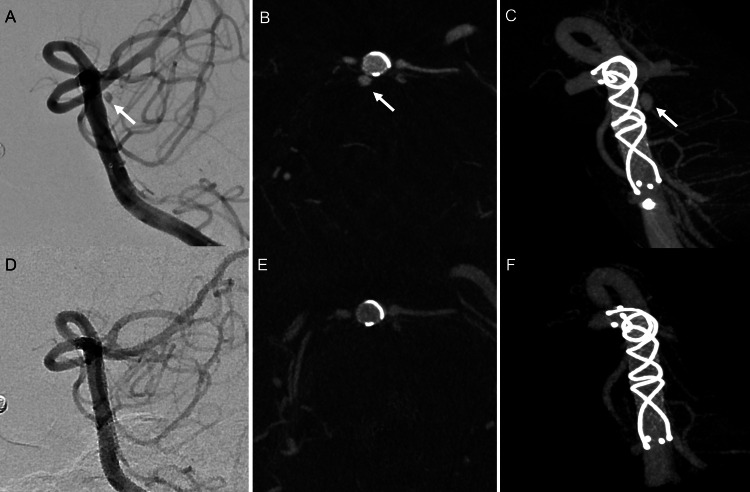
Left vertebral artery angiography just after stent placement and four months after stenting. (A-C) Immediately after low-profile visualized intraluminal support (LVIS) stent placement for the basilar artery blood-blister aneurysm (BBA). (A) The BBA was visualized (arrow). Left vertebral artery digital subtraction angiography at the working angle. (B) Cone-beam CT axial image and (C) 3D maximum intensity projection image. (D-F) Four months after stenting, the BBA had been completely obliterated. Panels D-F correspond to A-C, respectively.
